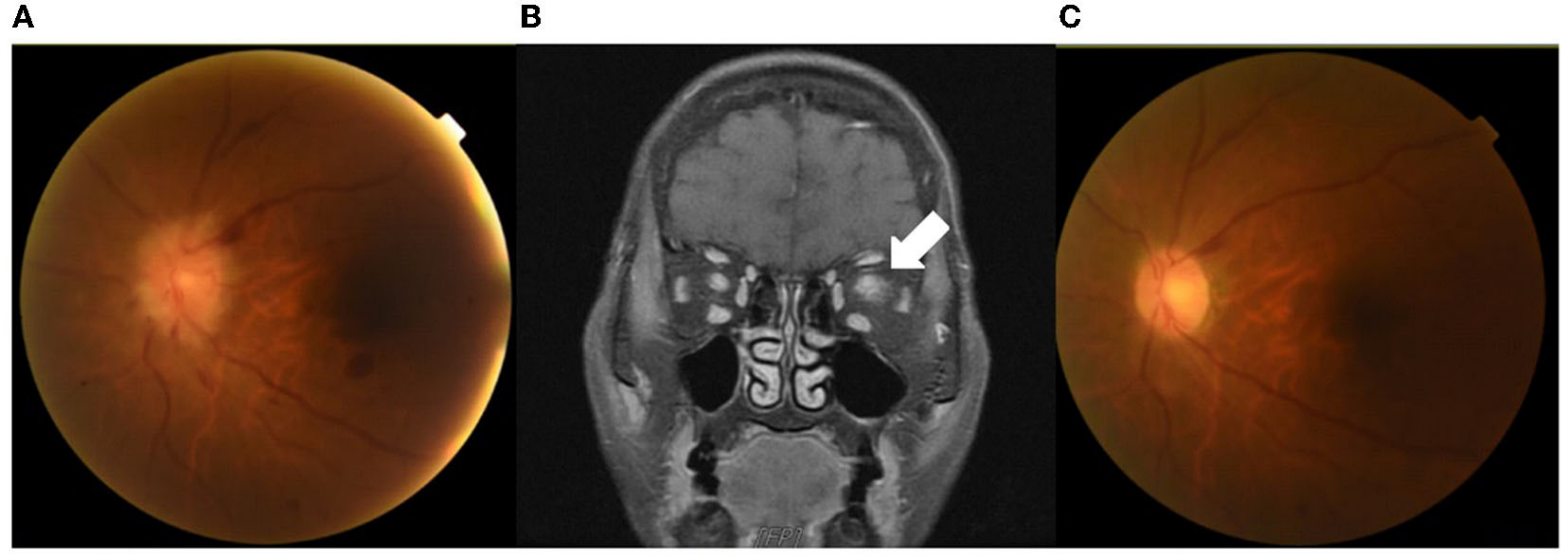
Images of contrast MRI and ophthalmoscopy. An ophthalmoscopic examination on Day 18 showed left optic disk papilledema with retinal hemorrhage (A). Brain MRI after gadolinium administration on the same day showed swelling of the left optic nerve with increased enhancement (arrow), compatible with optic neuritis (B). An ophthalmoscopic examination on Day 45 showed the resolution of papilledema and retinal hemorrhage (C).
